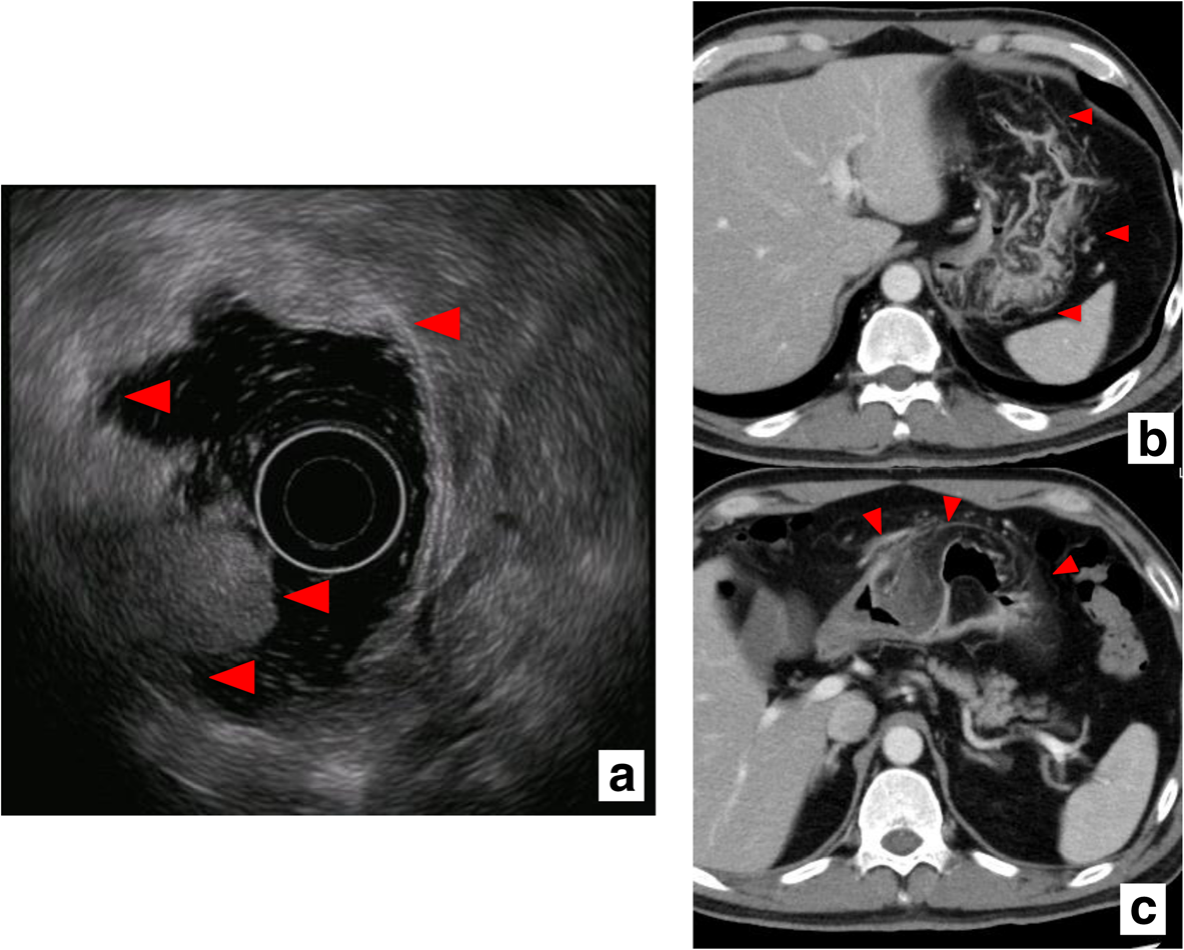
Findings on EUS and CT. EUS (a) and CT (b, c) findings are shown. EUS shows a high-echoic lesion in the antral wall submucosa (red triangle), extending to the stomach. CT shows a huge fat-containing mass lesion around the gastric wall, excluding the lesser curvature (red triangle). Abbreviations: CT computed tomography, EUS endoscopic ultrasound
